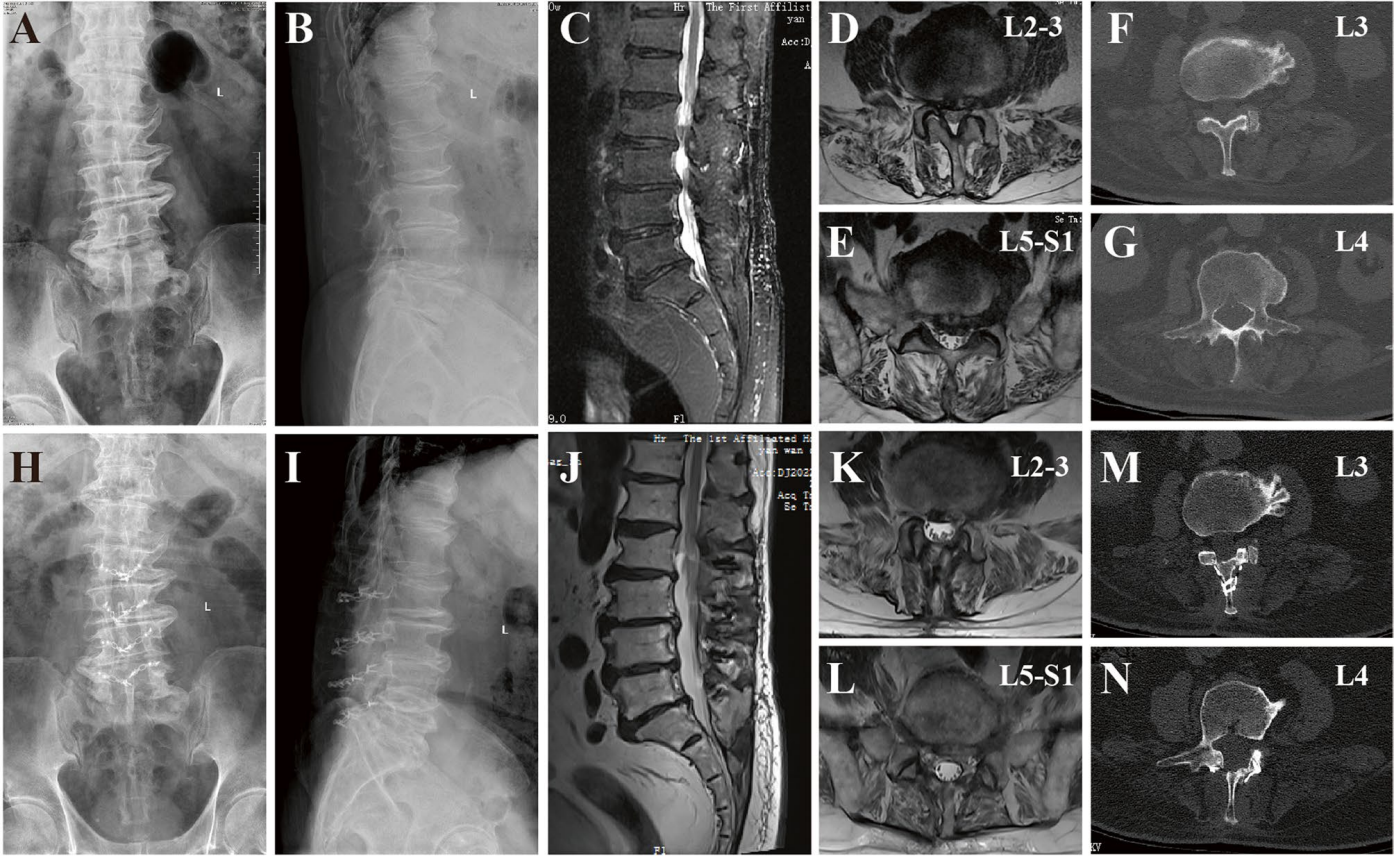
Representative case. A 74-year-old male with 5-level lumbar canal stenosis (LCS) underwent lumbar laminoplasty. Pre-operative imaging (A-G) includes X-rays (A, B) demonstrating extensive osteophyte formation, an MRI (C) showing LCS at five levels with axial views confirming neural compression at L2-3 (D) and L5-S1 (E), and CT axial views revealing spinal canal narrowing at L3 (F) and L4 (G). Post-operative imaging at 26 months (H-N) shows X-rays (H, I) of the laminoplasty fixation, an MRI (J) demonstrating significant neural decompression with axial views at L2-3 (K) and L5-S1 (L) confirming resolution of compression, and CT axial views (M, N) revealing bony regrowth/reunion within the laminoplasty grooves at L3 and L4 levels
